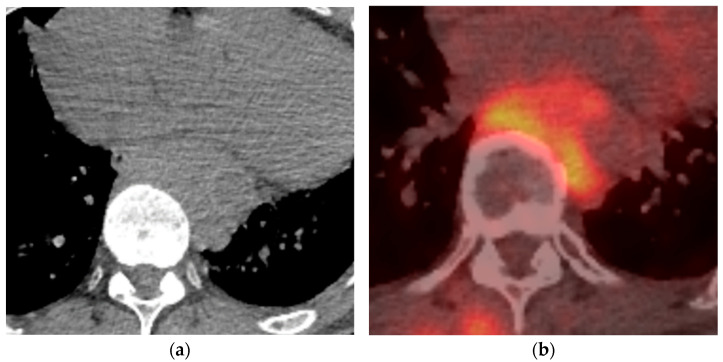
Biopsy-proven Mediastinal EP, case from our institution. (a) Unenhanced axial CT shows a posterior mediastinal soft tissue mass; (b) Axial PET-CT image shows heterogenous increased FDG-uptake in the posterior mediastinal mass.
